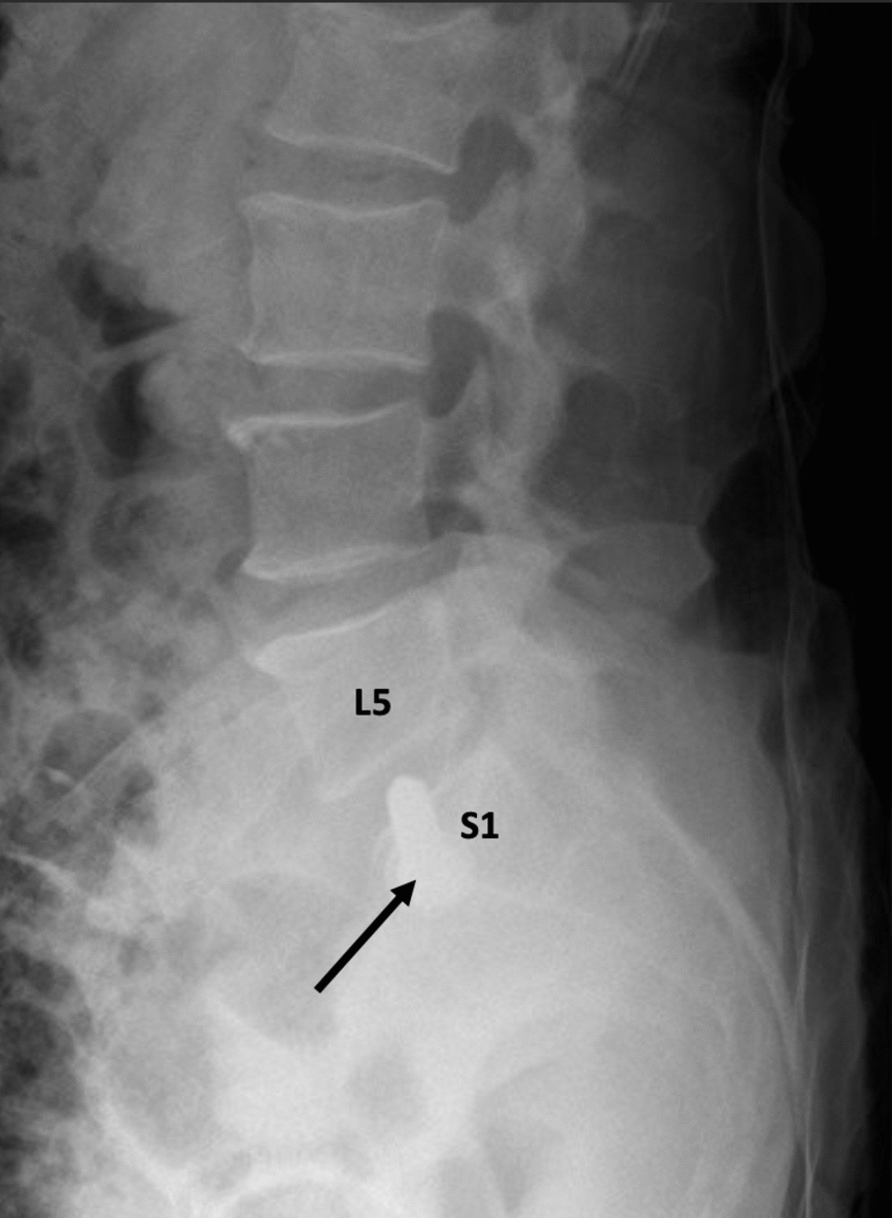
X-ray of the lumbar spine and the sacrum of the patient. L5: fifth lumbar vertebra, S1: first sacral vertebra. The arrow points to the misplaced ISs
